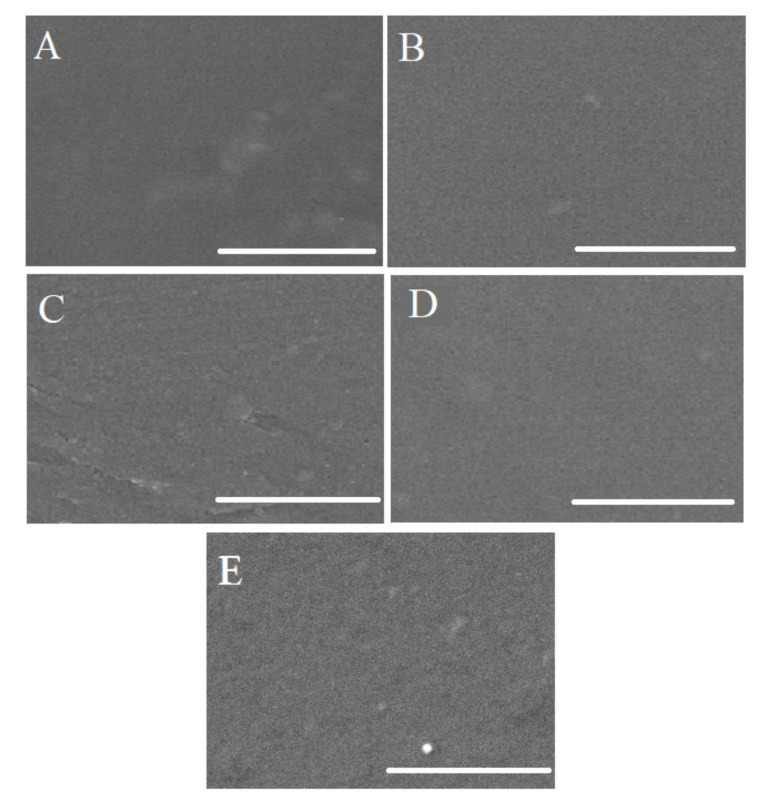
Surface morphology of (A) CTA:CDA (100:0), (B) CTA:CDA (0:100), (C) CTA:CDA (80:20), (D) CTA:CDA (60:40), and (E) CTA:CDA (50:50).
